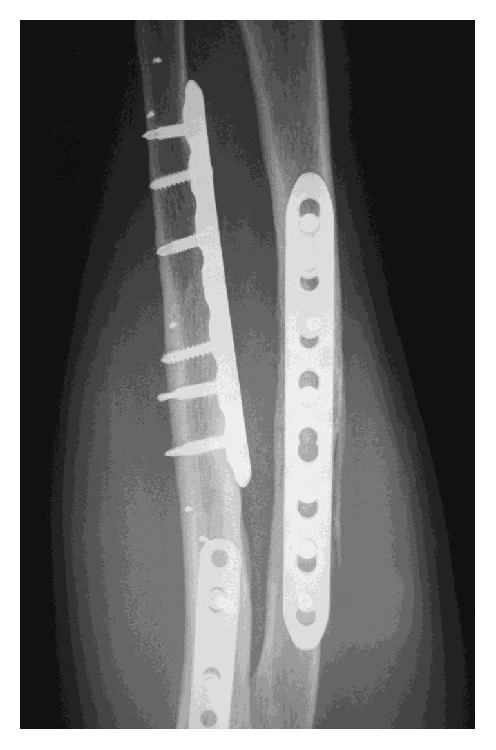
X-ray follow-up at 12 months after second surgery; there is no evidence of recurrence of bone formation.
